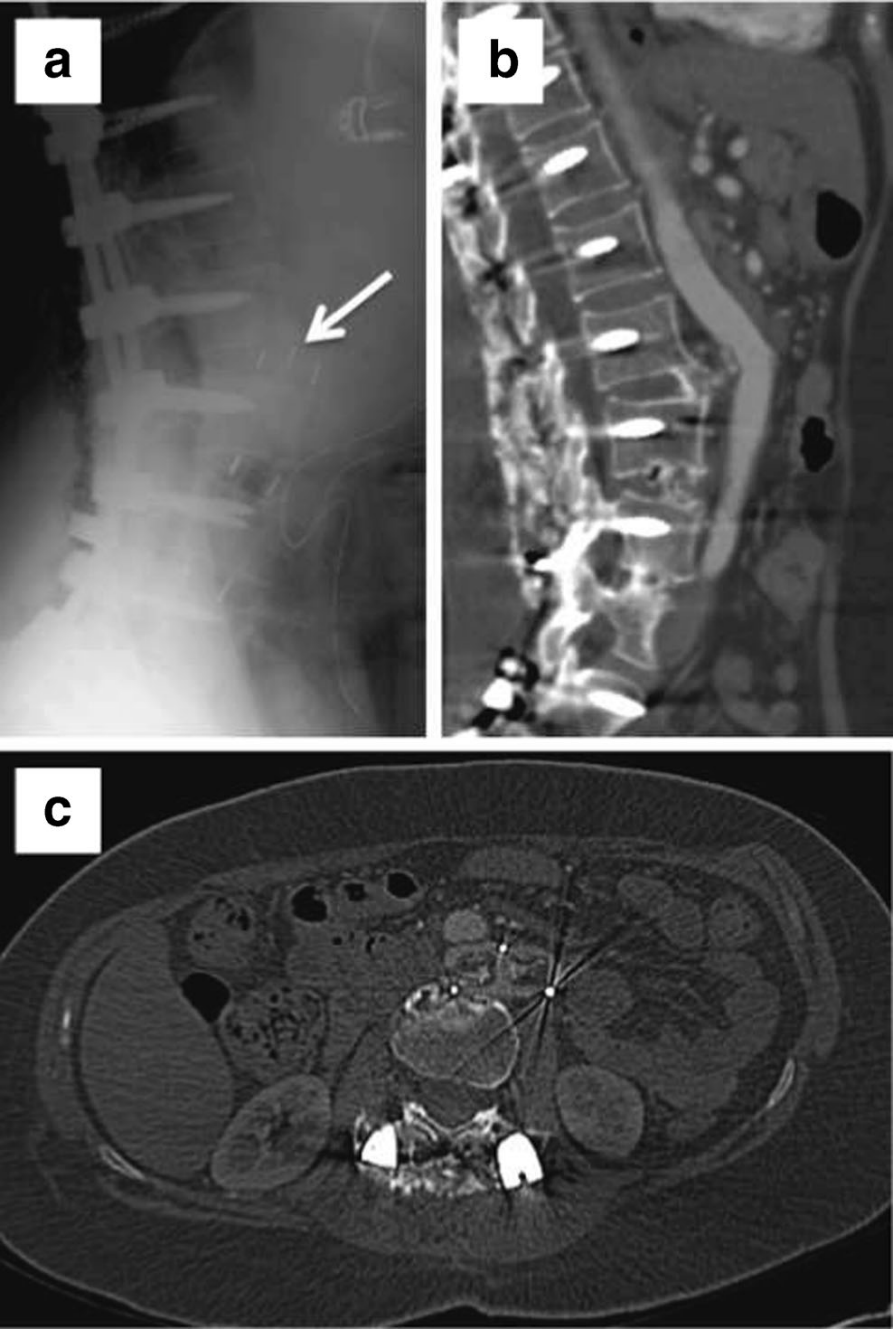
Lateral X-ray (a) shows anteriorly displaced spacer cage at L2–3. Sagittal (b) and axial CT (c) images show the cage indenting and displacing the abdominal aorta anteriorly better
